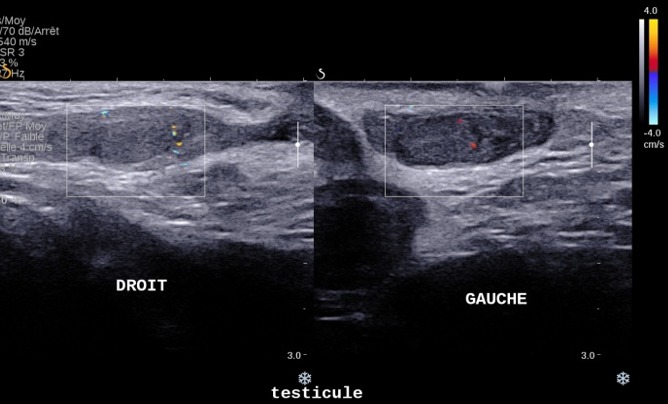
Testicule droit en position inguinale basse d’échostructure discrètement hypoéchogène faiblement vascularisé au doppler énergie: testicule gauche en intrascrotale
